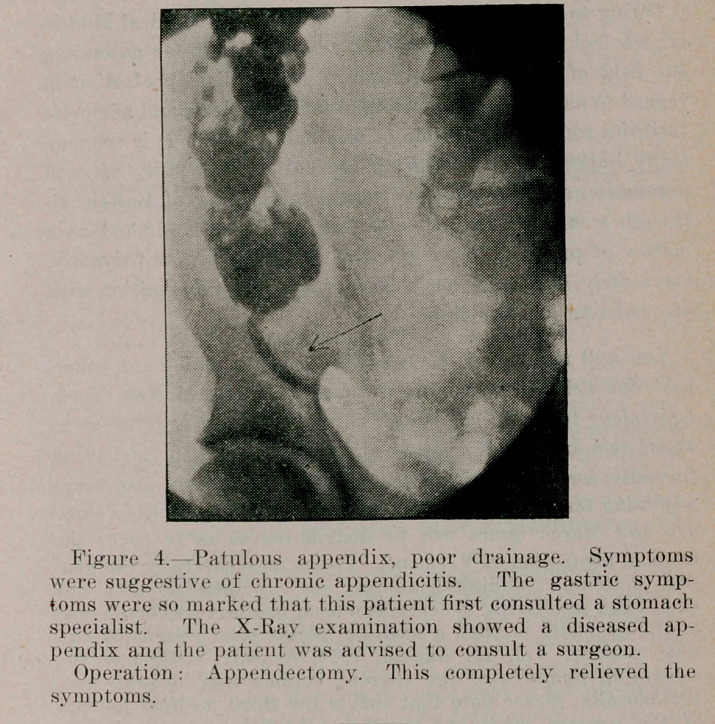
# X-Ray Diagnosis—Patulous Appendix
